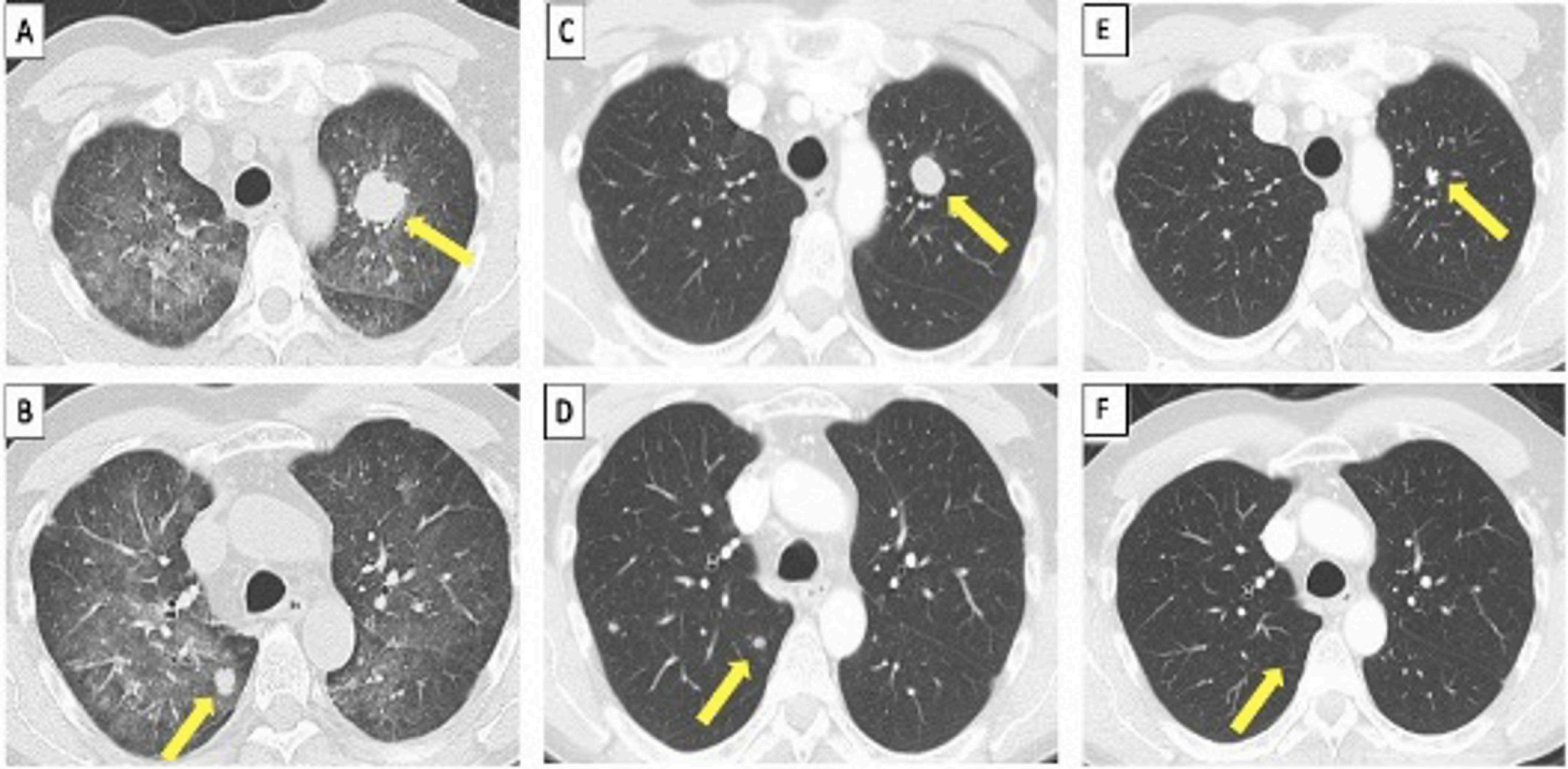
Comparison of the appearance of pulmonary metastasis and diffuse pneumonitis on CT scans. (A, B) In April 2017, multiple bilateral pulmonary metastases with superimposed ground glass opacities in the mid and upper lung fields. (C, D) In May 2017, resolution of diffuse pneumonitis and partial regression of lung nodules. (E, F) In February 2020, near-complete resolution of lung nodules.
